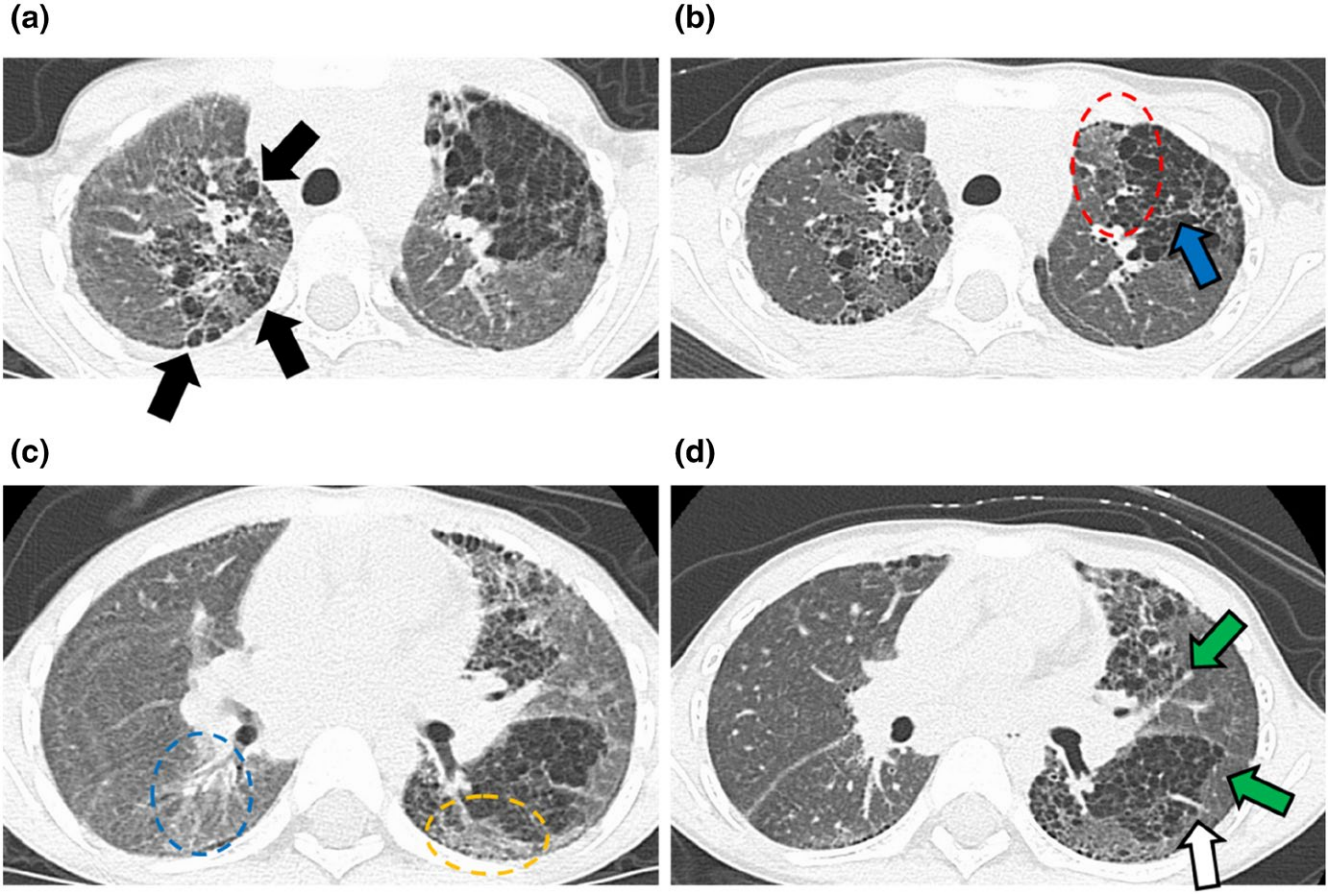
Tran‐axial thin‐slice CT images with 1‐mm thickness at the level of the aortic arch on the baseline (a) and followed up examination 6 month later (b), and the left atrium on the baseline (c) and followed up examinations (d) are demonstrated. In addition to the dorsal and mediastinal subpleural area of the right upper lobe (black arrows), air‐trapping area in the left upper segment seen on the baseline examination diminished mildly on the followed‐up examination especially for the inner area (red dotted circle). A pulmonary vessel in the left upper air‐trapping area got enlarged, and its border become distinct on the followed‐up examination (blue arrow). Compared with the baseline examination, mild decrease in the air‐trapping area in the left lingular and lower lobes was observed on the followed‐up examination (green arrows), with slight enlargement in pulmonary vessel inside the air‐trapping area in the left lower lobe (white arrows). These changes in image finding indicates the improvement in peripheral bronchial lesions and are very important. In addition, faint ground‐glass attenuation area in the right lower lobe (blue dotted circle) as well as subpleural reticular lesion (orange dotted circle) detected on the baseline examination almost disappeared on the followed up one.
